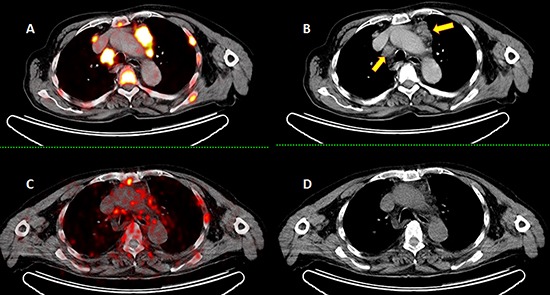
Ga-PSMA PET images A&B. of a 72-year-old patient with diffuse bone and lymph node metastases (yellow arrows show the enlarged lymph nodes) received two cycles of Lu-PSMA. Ga-PSMA PET images C&D. 2 months after the second cycle show a very good response with reduction in PSMA expression as well as a size reduction of the lymph node metastases in CT B&D.
